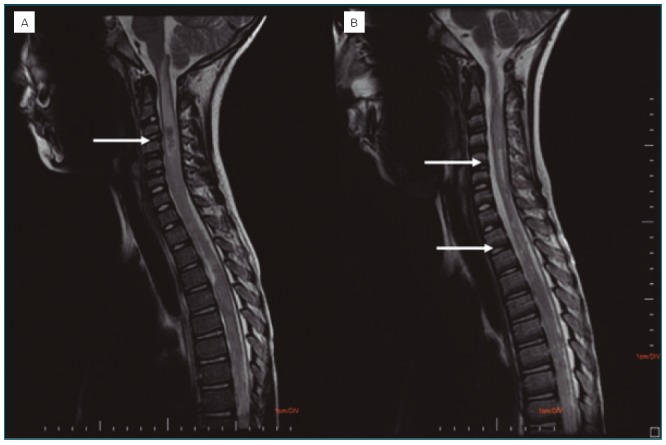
(A) MRI of the cervical spine at the time of diagnosis. The T2-weighted image shows an infiltrative formation with a tumefactive effect. (B) Control cervical spine MRI made after three months shows a reduction in swelling, the appearance of irregularities and tapering areas, and increments of intramedullary cystic degeneration foci.
